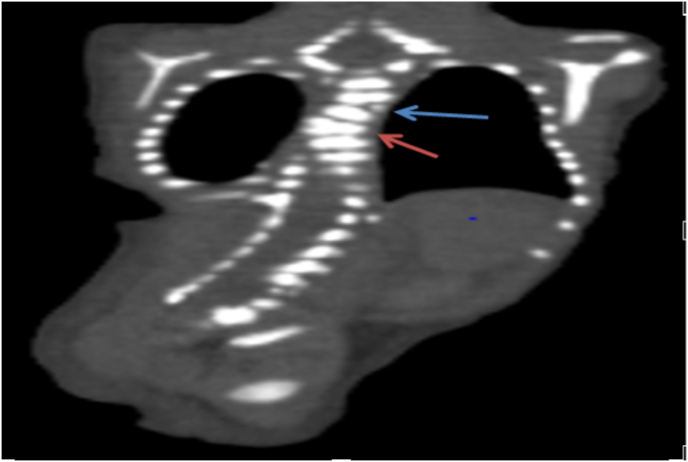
Coronal CT scan shows hemivertebra and scoliosis.
